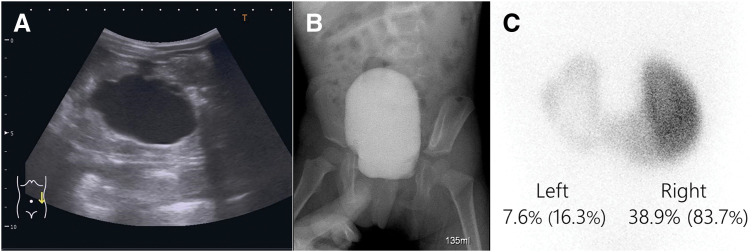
Preoperative examination images. (A) Ultrasonography showed dilation of the left renal pelvis and renal calyces (SFU classification grade 3–4). (B) Voiding cystourethrography showed no vesicoureteral reflux. (C) Renal scintigraphy revealed the HSK, and the DRF in the left kidney was 16.3%. DRF, differential renal function; HSK, horseshoe kidney; SFU, Society for Fetal Urology
